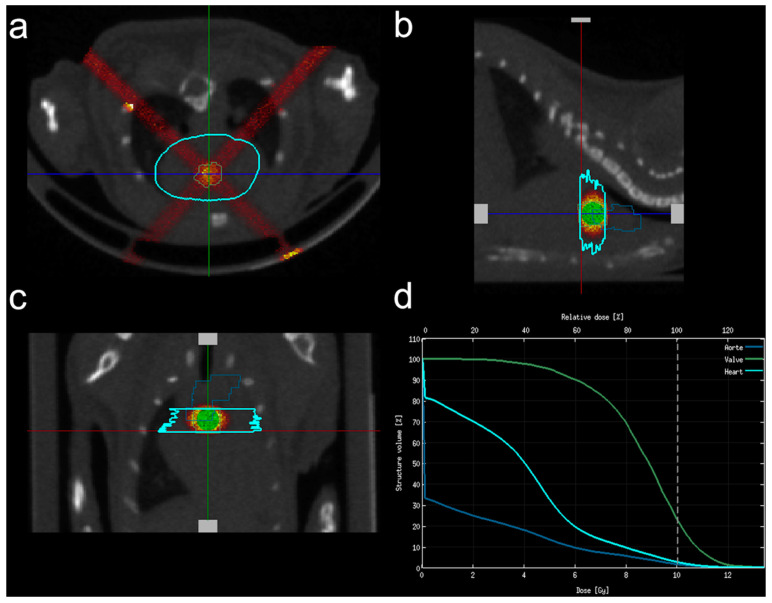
Irradiation plan consisted of 2 X-ray beams of 2 mm diameter with an angle of 45° and 135°. (a) Axial view; (b) sagittal view; (c) frontal view; (d) relative dose calculation within the aortic valve (green line), the surrounding heart (light blue) and the aortic arch (dark blue).
